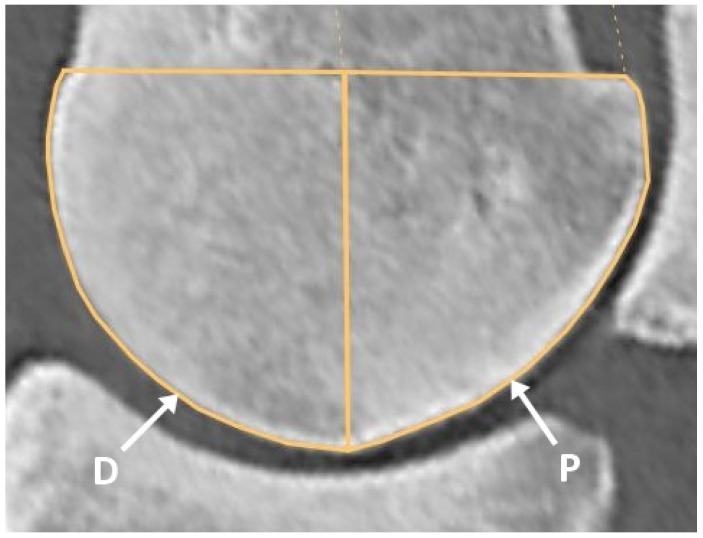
Sagittal plane computed tomographic reconstruction of the medial condyle of the third metacarpal bone, demonstrating the dorsal (D) and palmar (P) measurement regions, where mean Hounsfield Unit values were recorded. The midpoint of the line connecting the two most proximal points of the condyle was connected to its most distal point. The areas located dorsal and palmar to this line were then examined separately.
